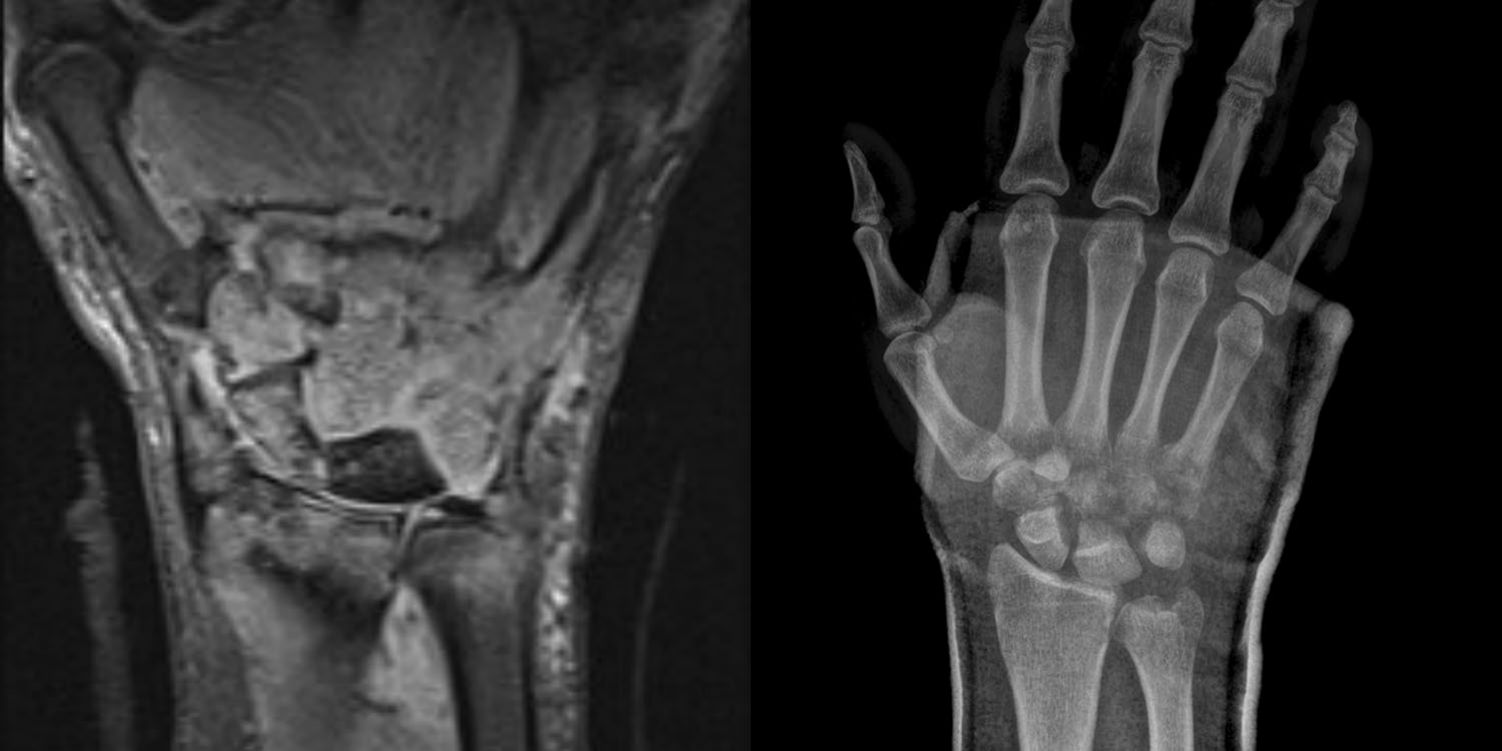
a MRI and b p/a radiograph at initial presentation in our department. Vast erosions of the wrist bones due to osteomyelitis. The MRI shows septic arthritis beginning in the bases of the metacarpal bones continuing proximally to the distal parts of radius and ulna
